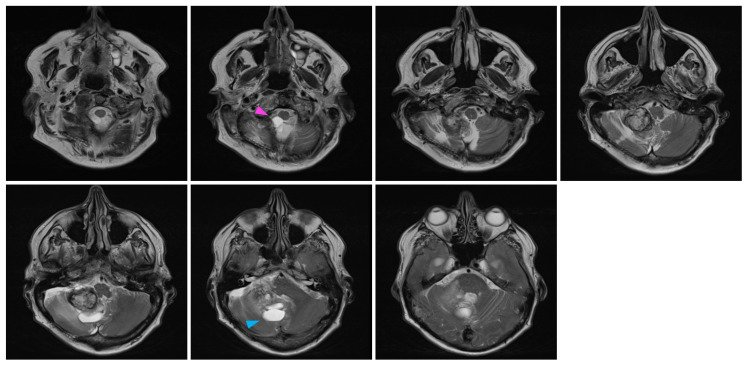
Pre-surgical MRI of the lesion. Representative capture of the T2-weighted MRI pre-surgical imaging highlighting the two cystic components of the lesion. Coronal images are organized left to right and represent a posterior-to-anterior progression in the coronal plane. The pink marker highlights a 21 mm antero-infero-medial cystic lesion, while the blue marker highlights a 32 mm posterior cystic lesion.
